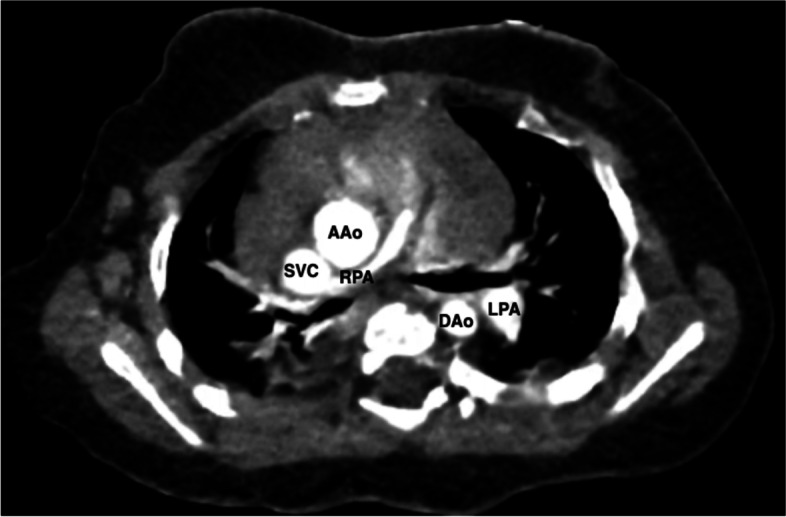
Preoperative computed tomography (CT). Axial images showed a hypoplastic right pulmonary artery (RPA) with a diameter of 3.8 mm
